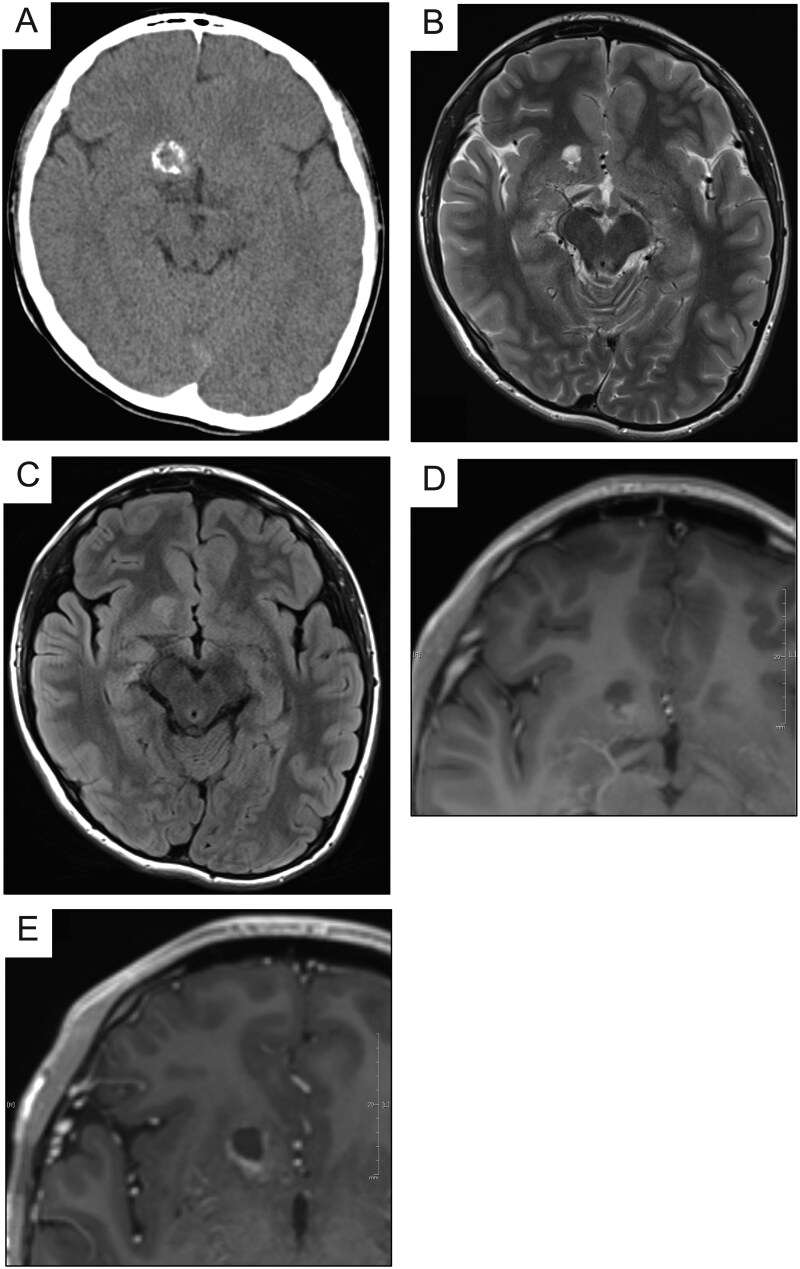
Details of imaging findings. (A) Computed tomography (CT) images at initial diagnosis showing a nodular lesion with heterogeneous calcification in the right basal ganglia. (B) T2-weighted magnetic resonance (MR) image showing a hyperintense lesion between the head of the right caudate nucleus and ventral putamen. (C) MRI fluid-attenuated inversion-recovery imaging (FLAIR) showing no peritumoral edema. (D, E) Gadolinium-enhanced T1-weighted MRI images showing heterogeneous enhancement at diagnosis (D) and tumor enlargement to ∼2.0 cm after 4 years (E).
